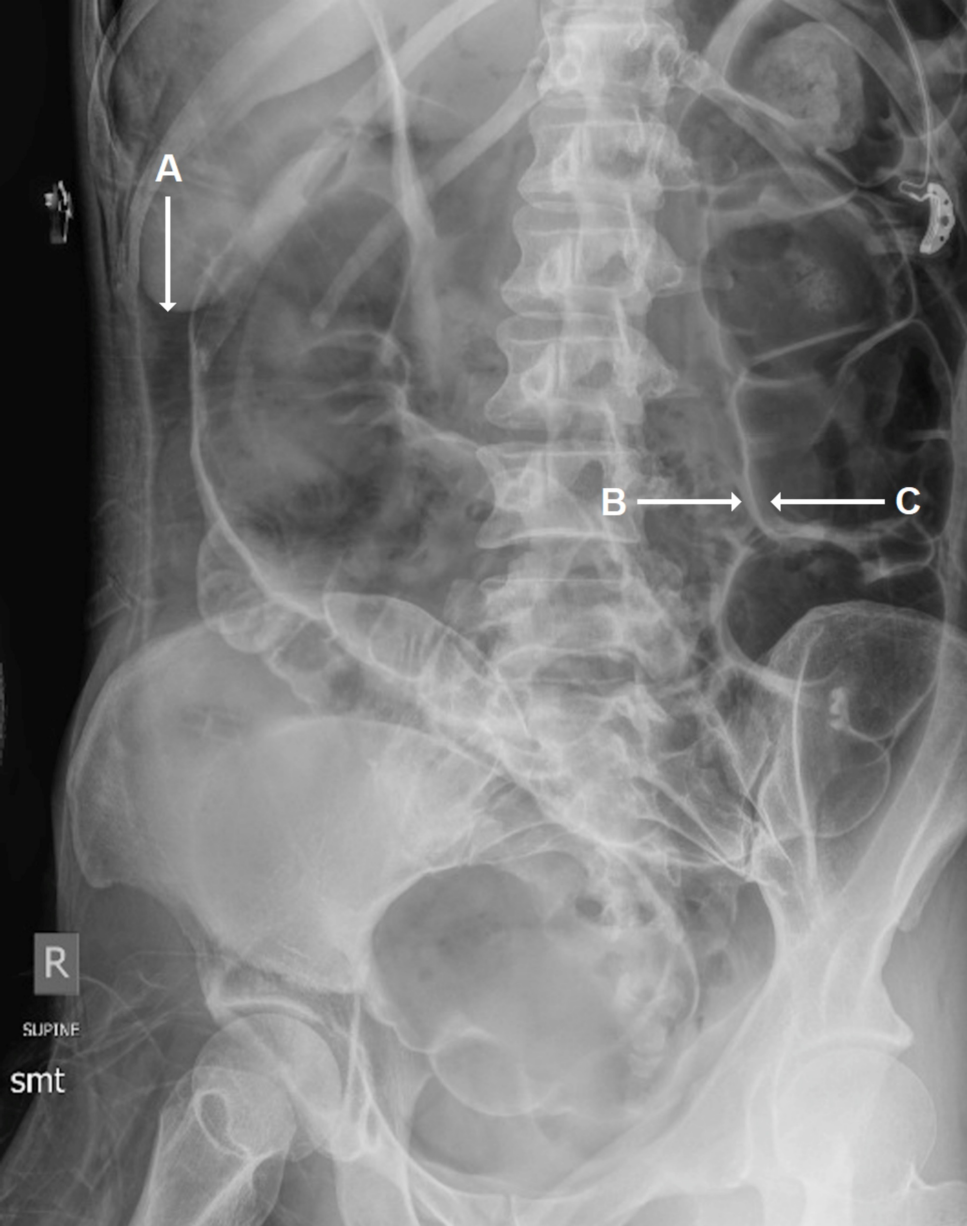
Abdominal Radiograph Abdominal radiograph depicting intraperitoneal air (A) and both peritoneal (B) and luminal (C) colonic wall air (Rigler’s or double wall sign).
